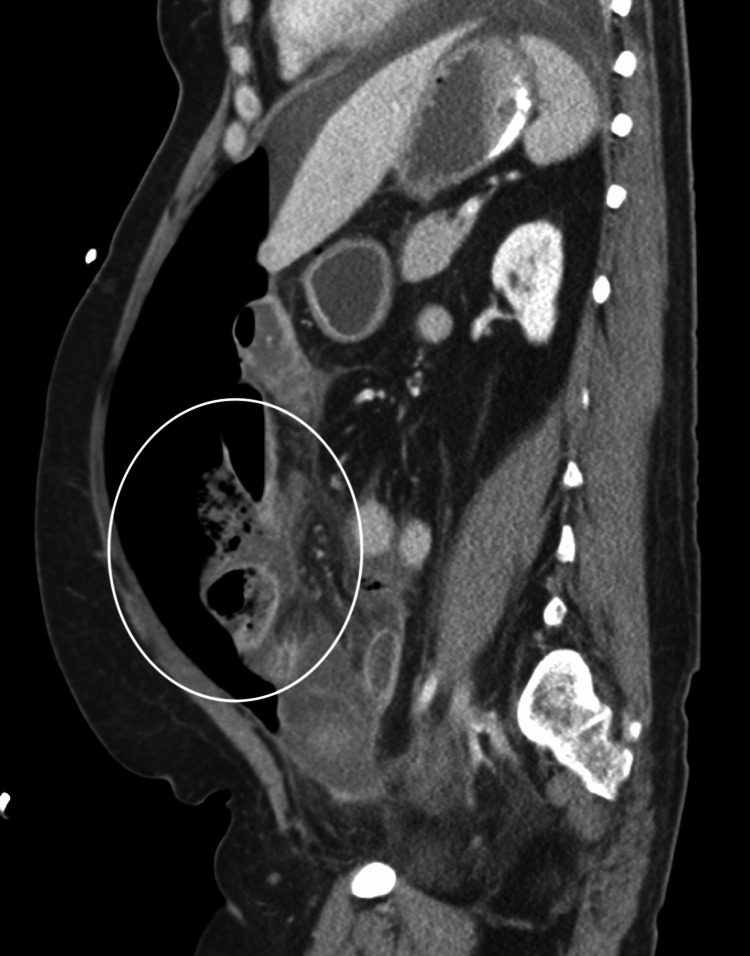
CT of the abdomen (sagittal view) showing pneumoperitoneum with significant abdominal distention.
